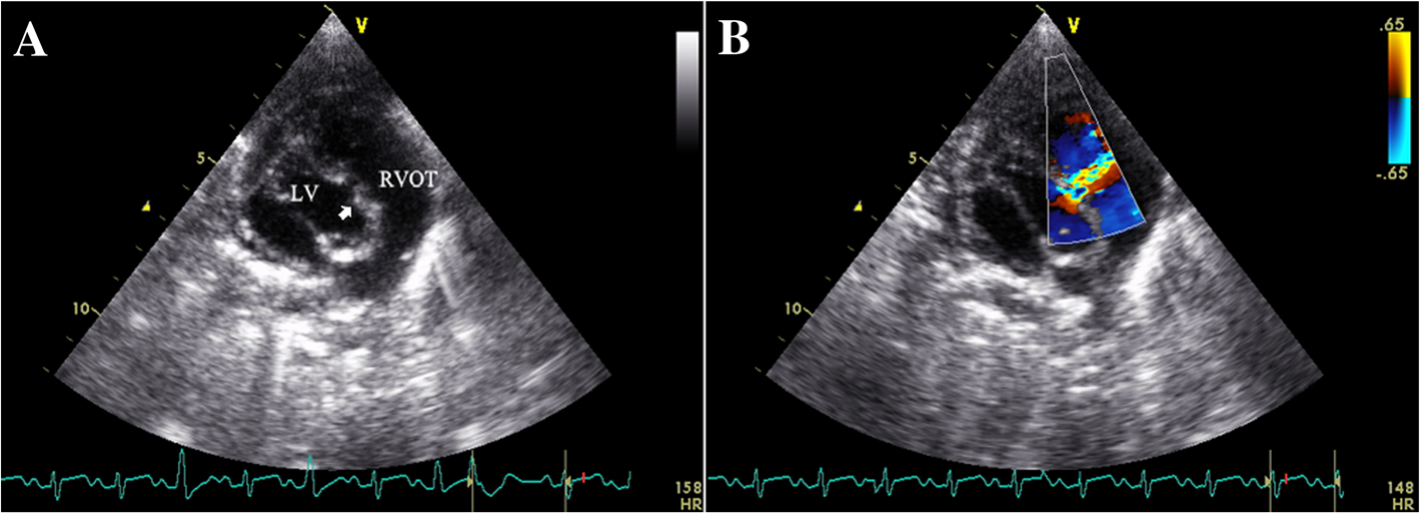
A TTE subcostal view showing the VSD. B The spectrum of Doppler imaging showing VSD.
